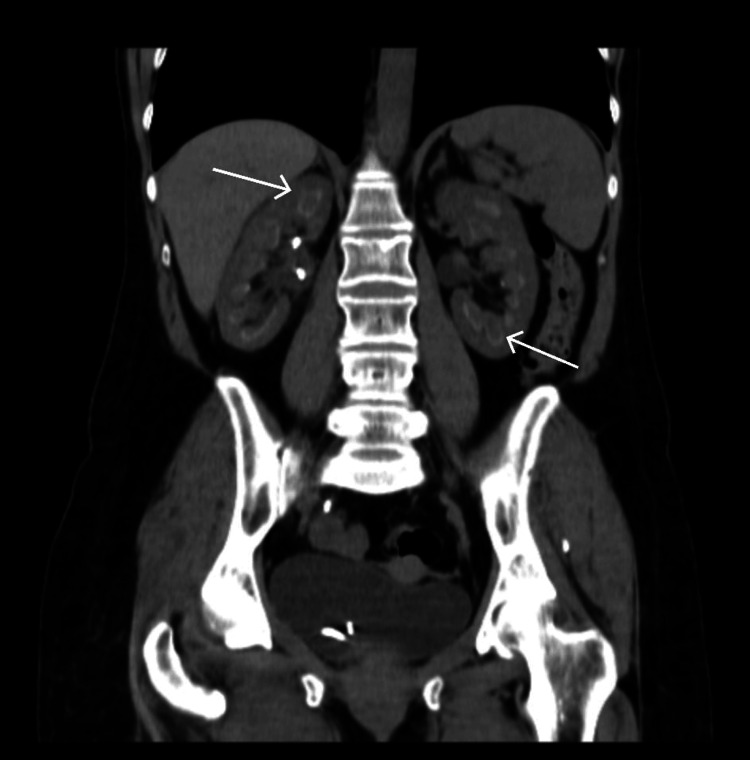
CT showing bilateral nephrocalcinosis (arrows)
